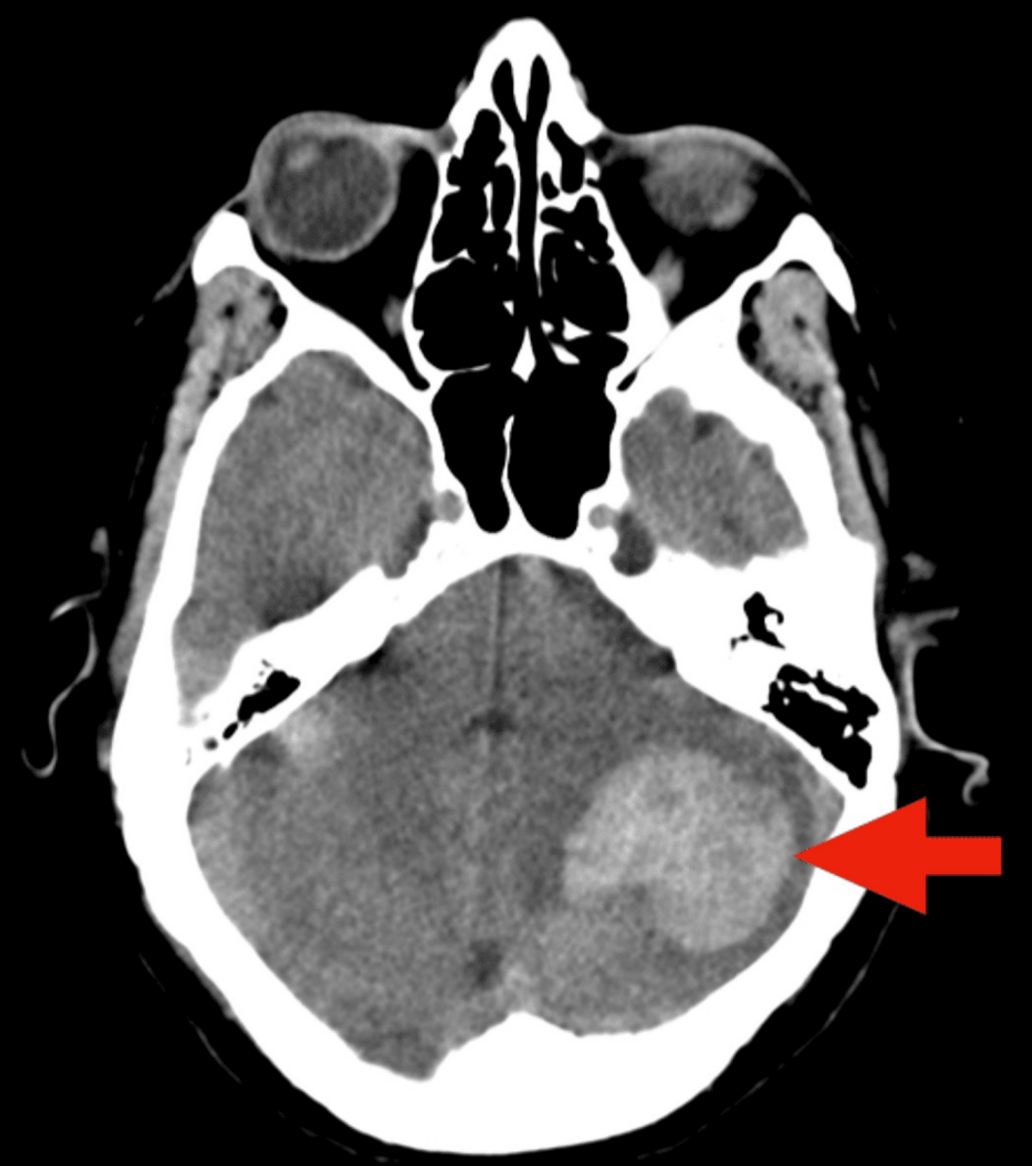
Non-contrast cranial CT scan CT scan showing spontaneous hyperdensity in the left cerebellar cortico-subcortical area compatible with hemorrhagic lesion (red arrow) CT: computed tomography
